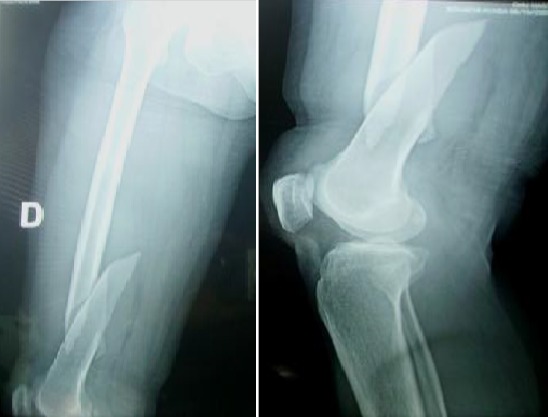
Radiographie préopératoire montrant une fracture de l’extrémité inferieure du fémur droit déplacée
